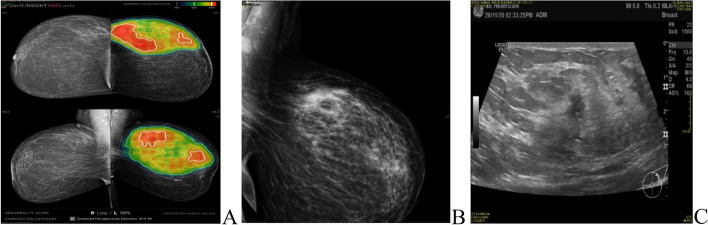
A, B 37-years-old female patient presented with Left breast UOQ extensive fine pleomorphic and amorphous calcifications of segmental distribution, with UOQ multiple indistinct irregular masses. C ultrasound showed left breast UOQ multiple irregular hypoechoic masses with calcific echogenic foci, the largest is seen at 1 o’clock measuring 13 × 15mm. Intraductal echogenic lesions are noted
